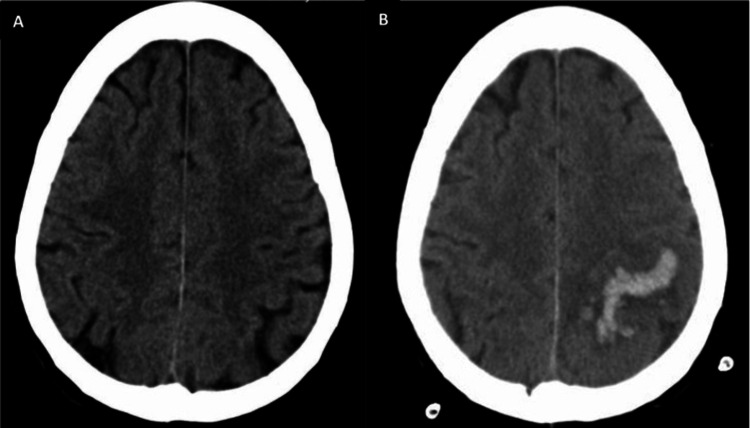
Cerebral CT findings. Initial cerebral CT showing no sign of any ischemic or hemorrhagic vascular event or any space-occupying lesion (A). Cerebral CT revealing a vascular lesion with a left rolandic cortico-subcortical hemorrhagic component and reduced amplitude of the regional sulci (B) CT: computed tomography
